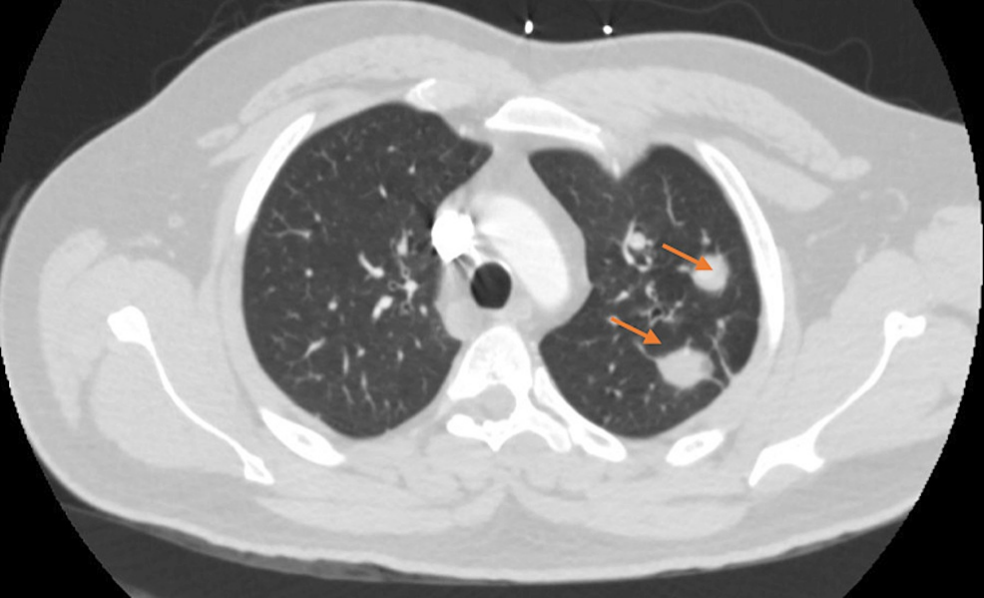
Axial view of CT angiogram pulmonary demonstrating two large, closely clustered pulmonary nodules with large serpentine enhancing vessels within the posterior lateral left upper lobe. CT, computed tomography
